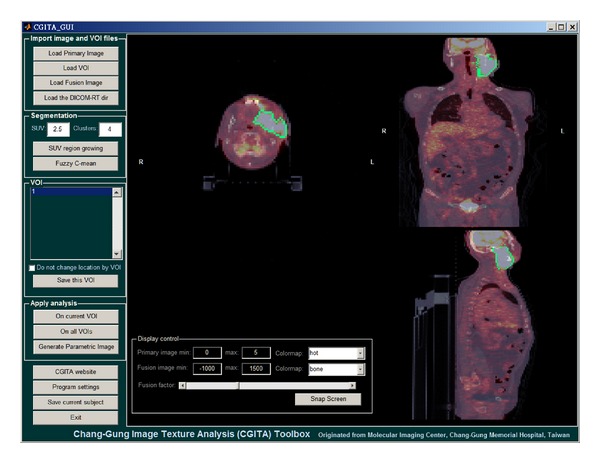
A screen shot of the CGITA program. The CGITA GUI provides users with a simple image display interface that allows users to examine different slices and views. The computation of heterogeneity indices is achieved simply by button clicking. As an open-source project, the current functions and interfaces of CGITA can be customized by users familiar with MATLAB programming. The screen shot here shows a subject with the FDG-PET images fused over CT images.
